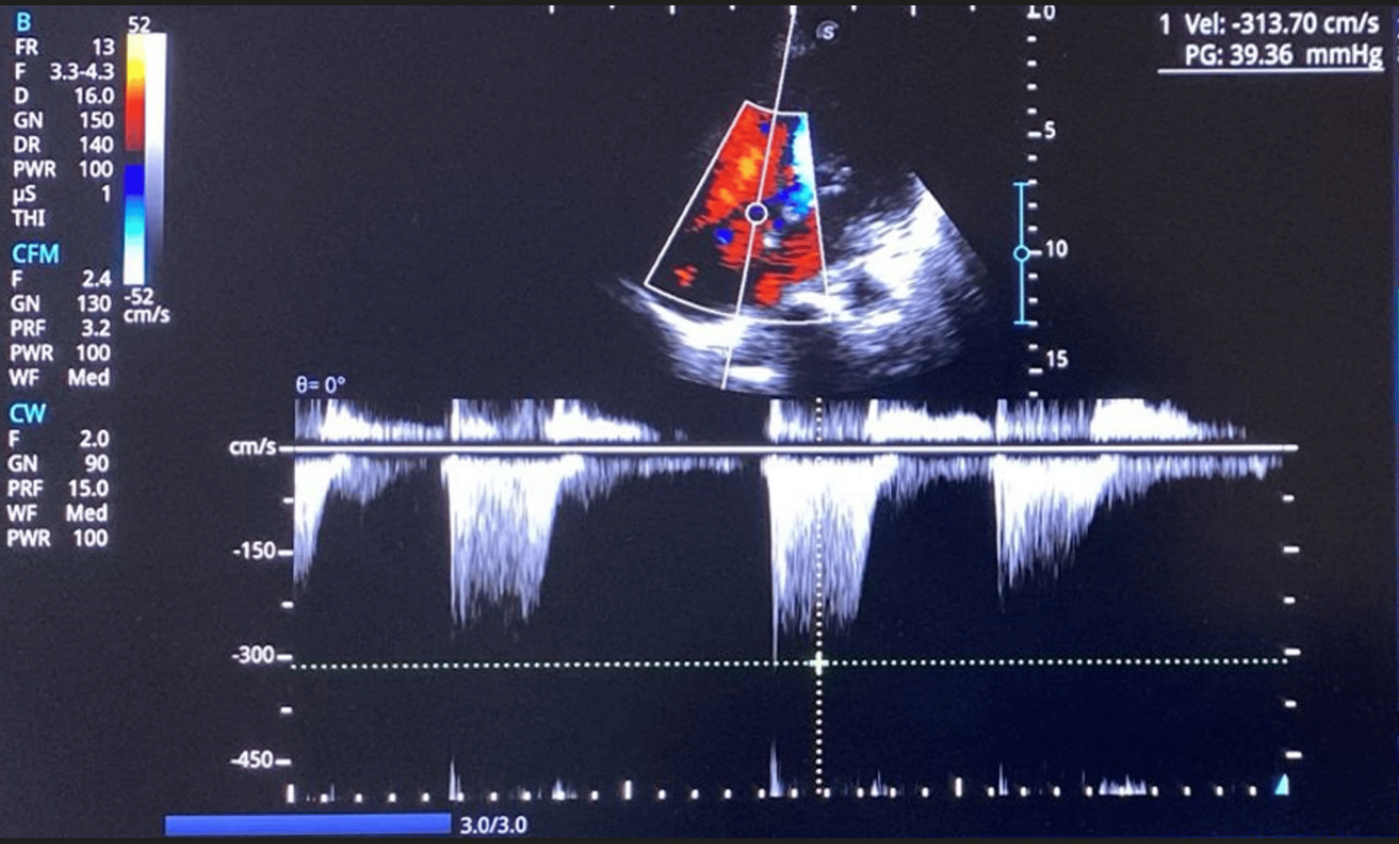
Echocardiography post-treatment (peak pressure gradient improved to 39 mmHg)
